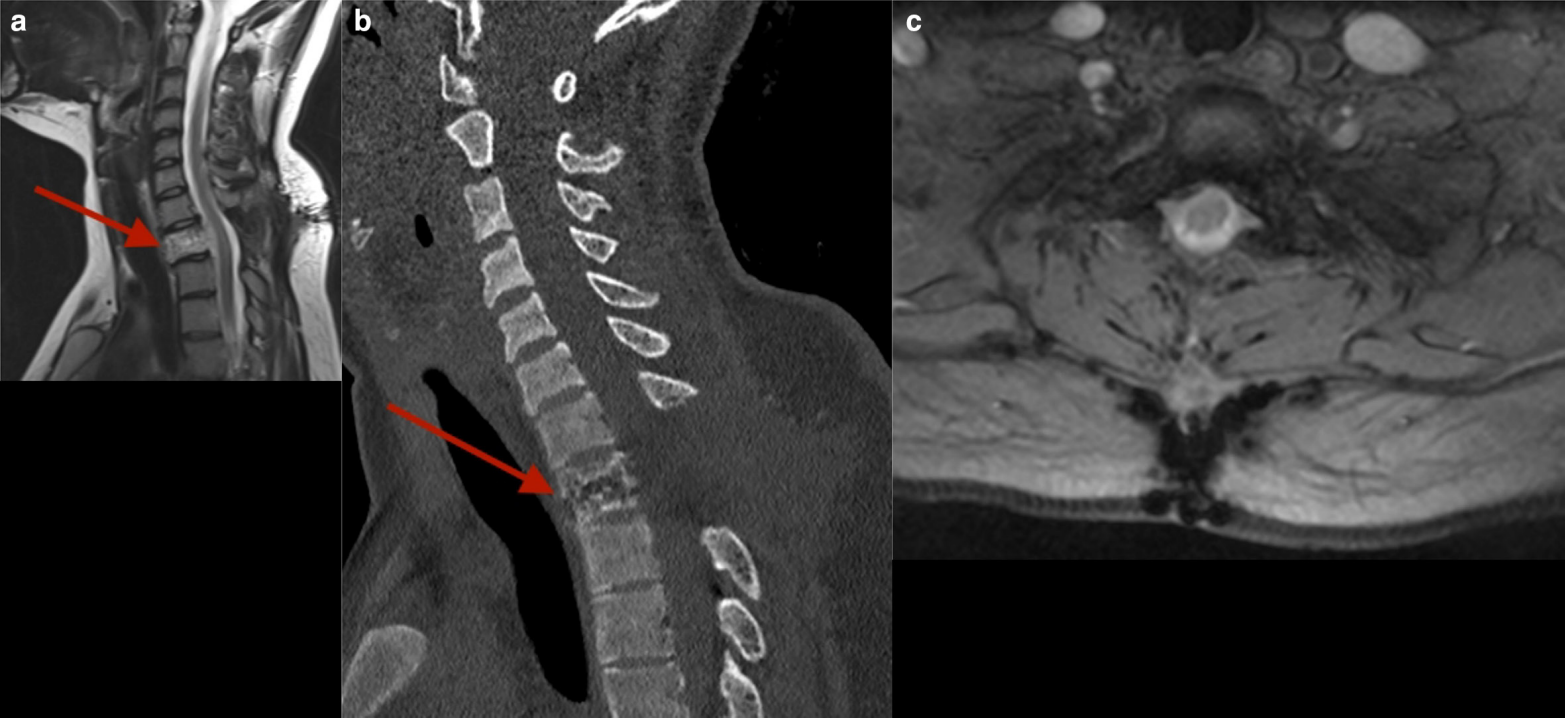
a. Postop sagittalT2 A sagittal slice from a *T*_2_-weighted sequence demonstrating the partially resected hyperintense haemangioma ( arrow) in the T1 vertebral body with resolution of cord compression following laminectomy b: Postop sagittal CT A sagittal image from a CT scan demonstrating the haemangioma and laminectomy at the level of the T1 vertebral body ( arrow). c: Postop Axial T2 gradient echo images An axial slice from a fat-saturated sequence highlighting the laminectomy at the level of the T1 vertebral body.
